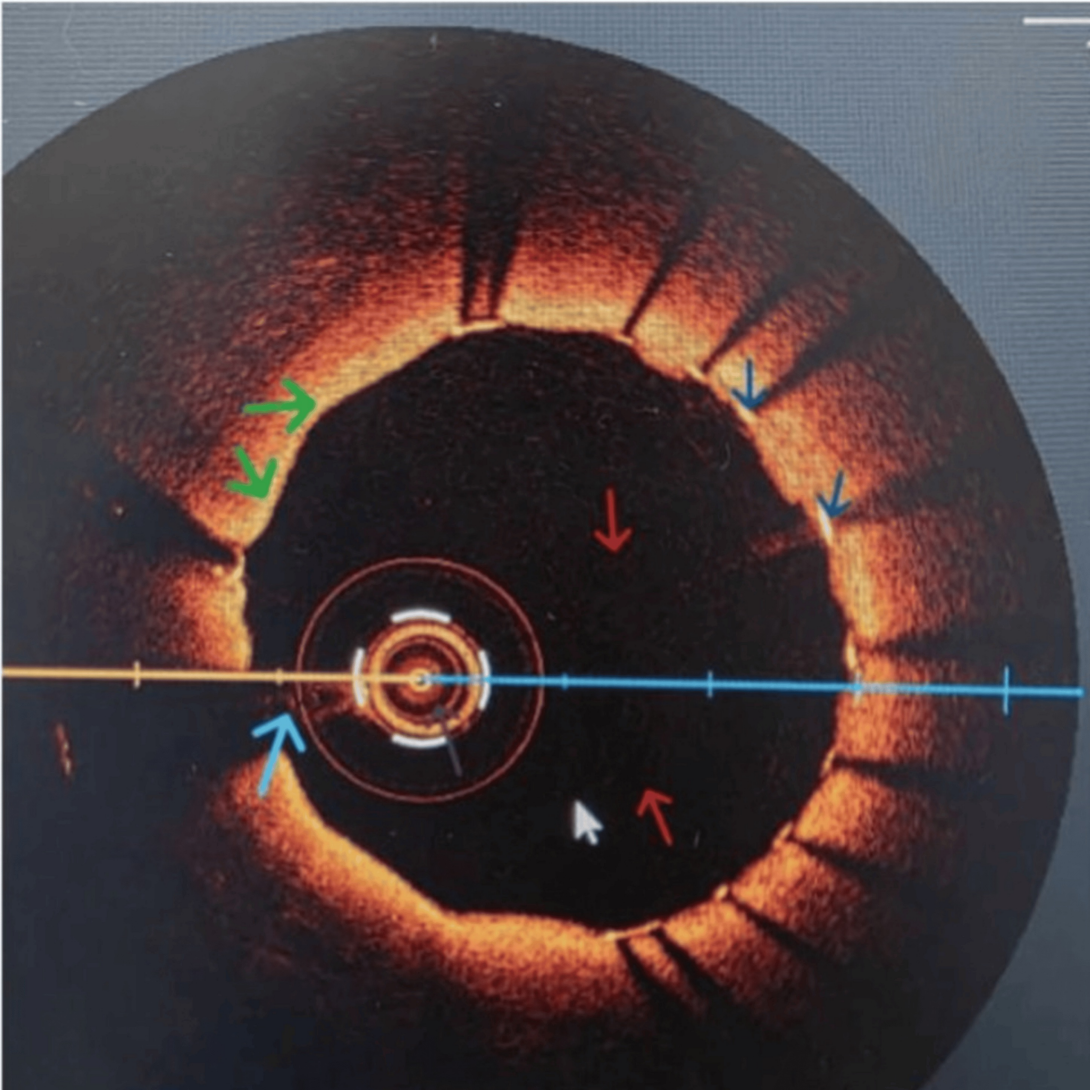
Another OCT image after stent insertion Dark blue arrows depict a stent with deflected comet-like shadows; red arrows show IVUS lumen devoid of blood; green arrows depict a ballooned lesion now buried within the intima with the DES, and the light blue arrow shows the guide wire DES: drug-eluting stent; OCT: optical coherence tomography; IVUS: intravascular ultrasound
